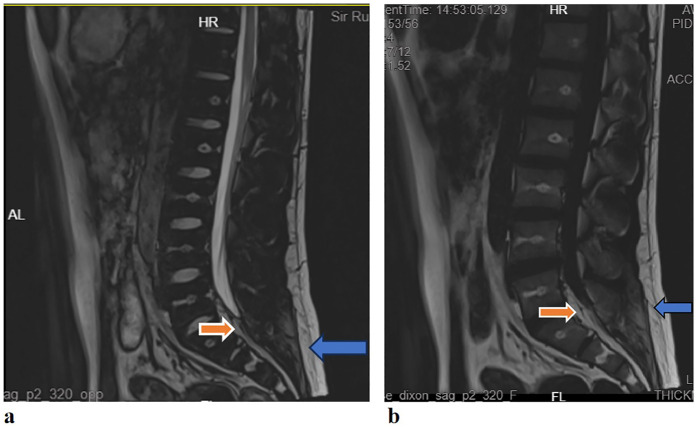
(a) Lumbar sacral MRI (T2WI): the sagittal plane showed a high signal behind the L5/S1 cone in the spinal canal (red arrow), which was the same as the fat signal (blue arrow); (b) lumbar sacral MRI [T2 fluid attenuated inversion recovery (FLAIR)]: the sagittal plane showed high signal behind the L5/S1 cone in the spinal canal (red arrow), which was the same as the fat signal (blue arrow).
